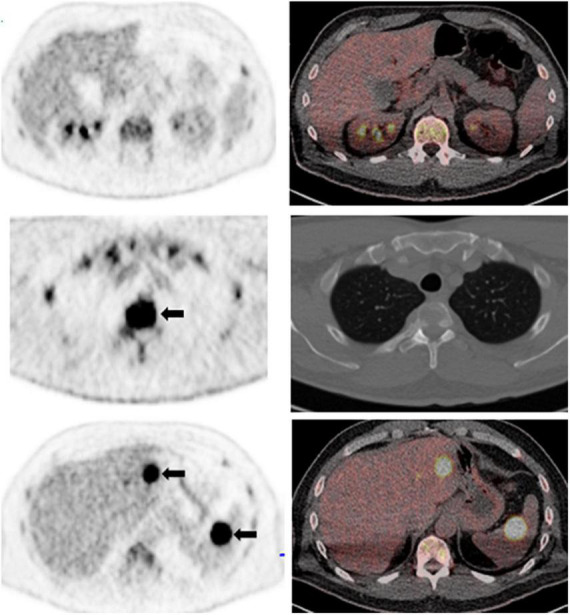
A 62-year-old man with a history of oligosecretory multiple myeloma. Whole body maximum intensity projection PET image (not shown) showed diffuse increased FDG uptake in the bone marrow above background liver (bone marrow Deaville score, 4). Representative axial PET (left column) and fused PET/CT images (right column) show diffuse increased bone marrow activity above liver (top row); and intensely metabolically active skeletal lesions (T3 vertebral body; arrows, middle row). There are also multiple metabolically active extraskeletal focal lesions with intense FDG uptake in the liver and spleen (arrows, bottom row); focal lesion Deauville score, 5.
